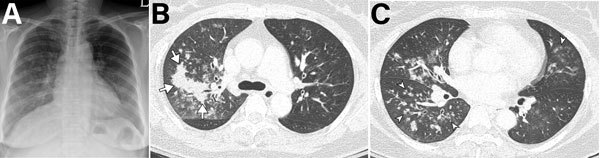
Imaging of 55-year-old immunocompetent woman with human metapneumovirus pneumonia, South Korea. A) Initial chest radiograph showing ill-defined patchy and nodular ground-glass opacities in the right lung and left lower lung zone. B, C) Chest computed tomography showing irregular nodular consolidation (arrows in panel B) and multiple ill-defined centrilobular nodular opacities (arrowheads in panel C) with mild bronchial wall thickening. Five days later, the lesions had resolved completely.
